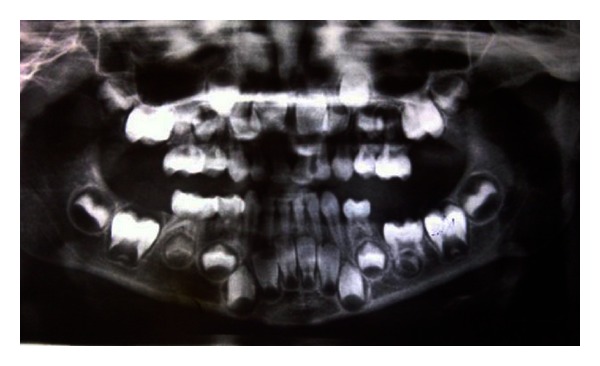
Radiographic view of the left second submerged primary mandibular molar.
